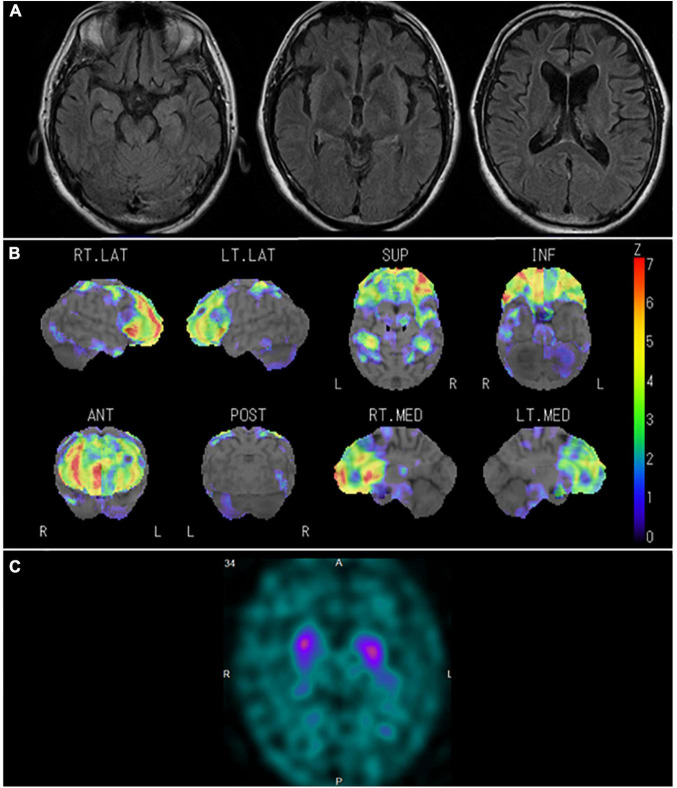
Neuroradiological findings in Case 1. (A) Magnetic resonance imaging shows marked bilateral frontal lobe atrophy. (B) Brain perfusion single photon emission computed tomography (SPECT) three-dimensional stereotactic surface projection analysis (Nihon Medi-Physics, Tokyo, Japan) shows significant bilateral frontal lobe hypoperfusion. The color scale for the Z score is shown on the right side of the figure. Presented in color if the Z-score was > 0. (C) Dopamine transporter (DAT) SPECT shows remarkably reduced DAT availability in the bilateral striatum.
